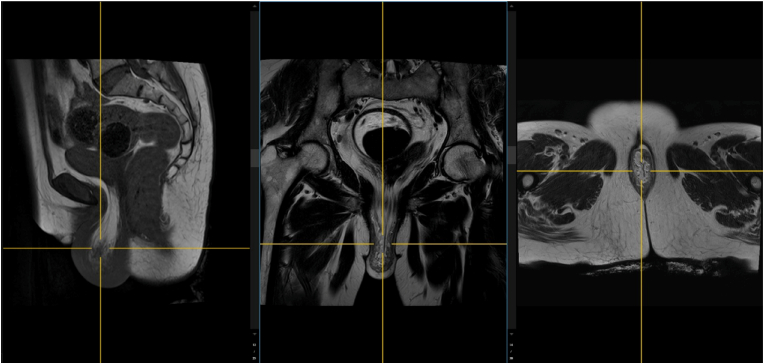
The abdominal MRI with contrast showed the prolapse of the bladder and perivesical fat, moderate uterine descent, mild anorectal junction descent, moderate hiatal enlargement, and severe pelvic floor descent.
